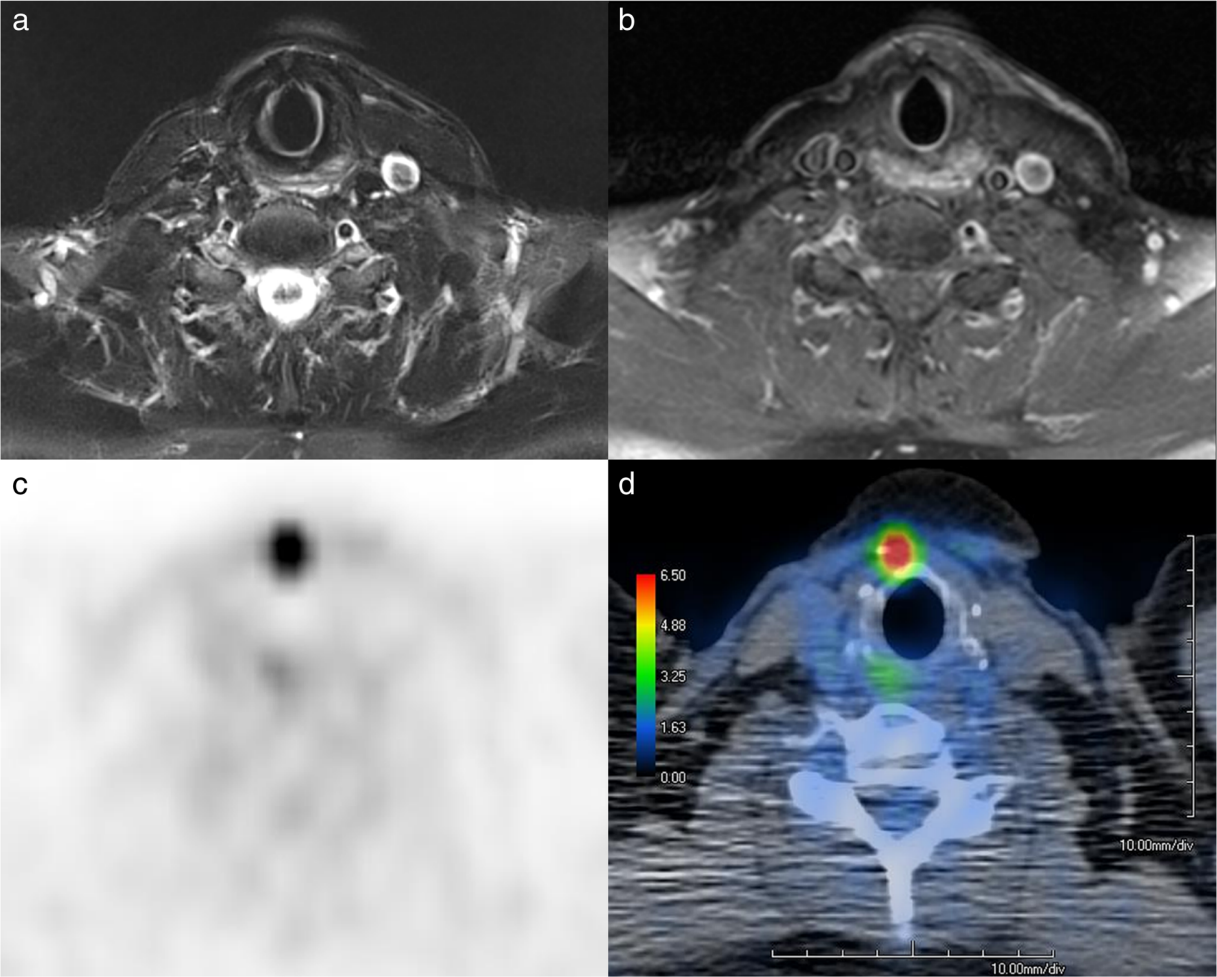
The added value of FDG-PET/ldCT for identifying a locally recurrent thyroid cancer with unspecific pretracheal finding via MRI. a STIR-weighted MR image, b contrast-enhanced T1-weighted MR image, c FDG-PET, and d fused FDG-PET and CT
